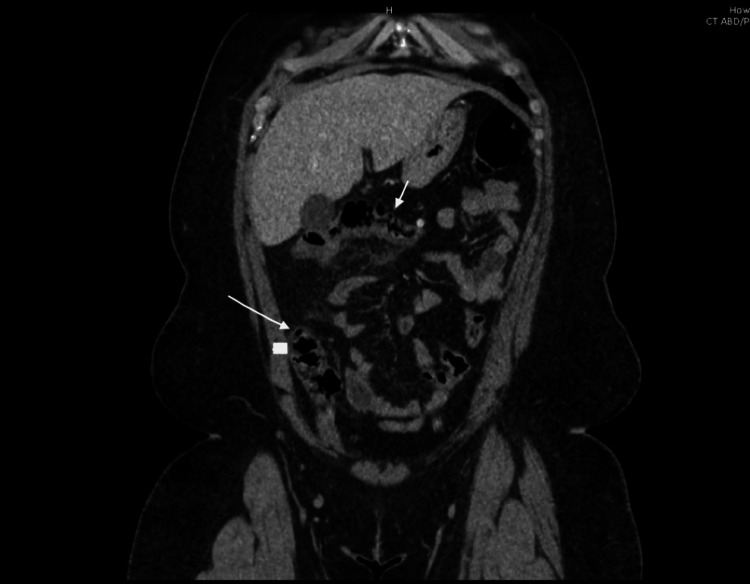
CT abdomen - coronal view CT of the abdomen with contrast showing diverticulosis in the transverse colon, hepatic flexure, and thickening of the hepatic flexure wall, accompanied by pericolonic fat stranding. The arrow points towards the diverticula.
